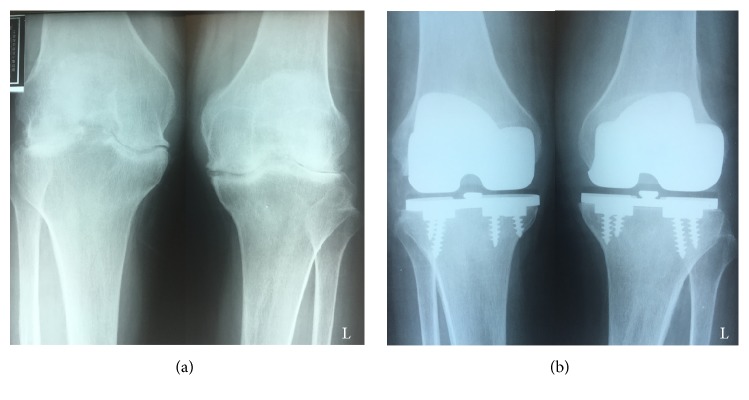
Plain radiographs of the bilateral knee of the patient with rheumatoid arthritis before and after Miller-Galante II Total Knee Arthroplasty performed 16 years earlier. (a) Preoperative plain radiograph shows bilateral rheumatoid arthritis of the knee categorized as Larsen grade 4. (b) Postoperative plain radiograph shows a femorotibial angle (FTA) of 174° on the right side and 183° on the left side. The left side shows varus malalignment.
